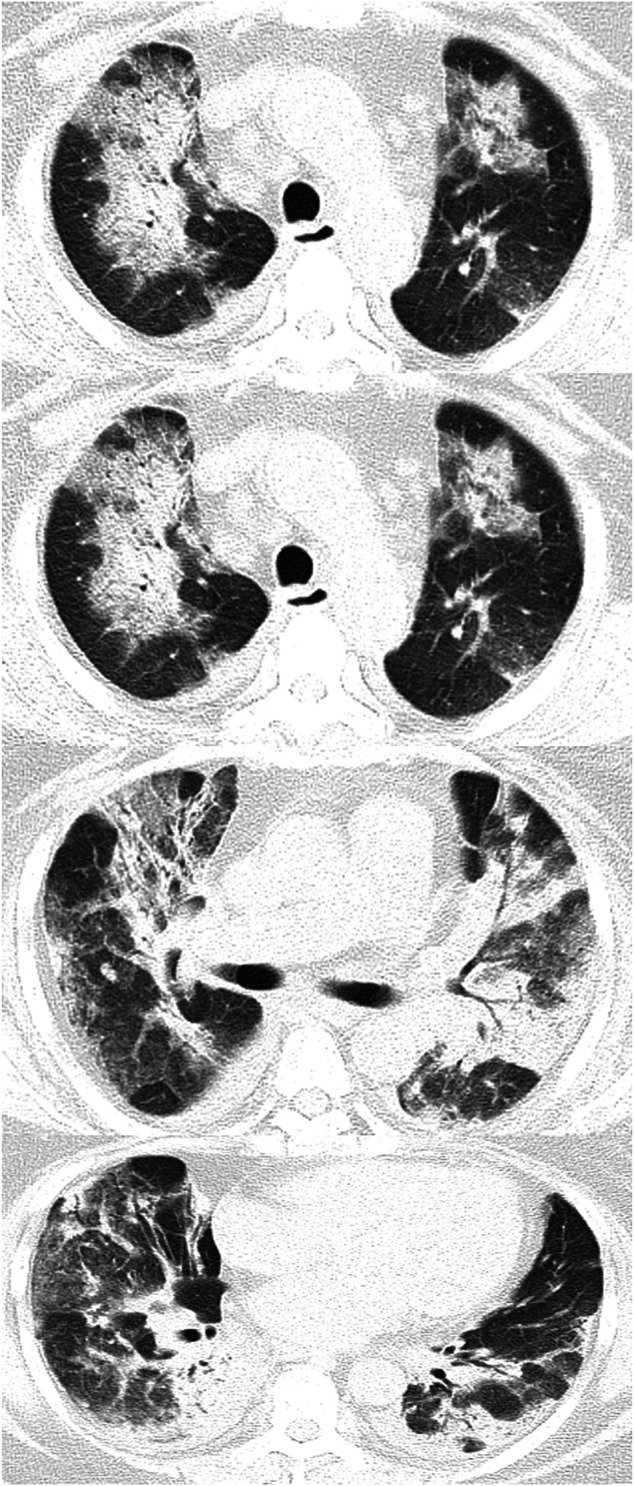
These scans are of a 64°year-old woman with an H1N1 infection confirmed by a sputum test. Chest CT scan demonstrates patchy shadows and ground-glass opacities in both lungs, with traction bronchiectasis and pleural thickening.
